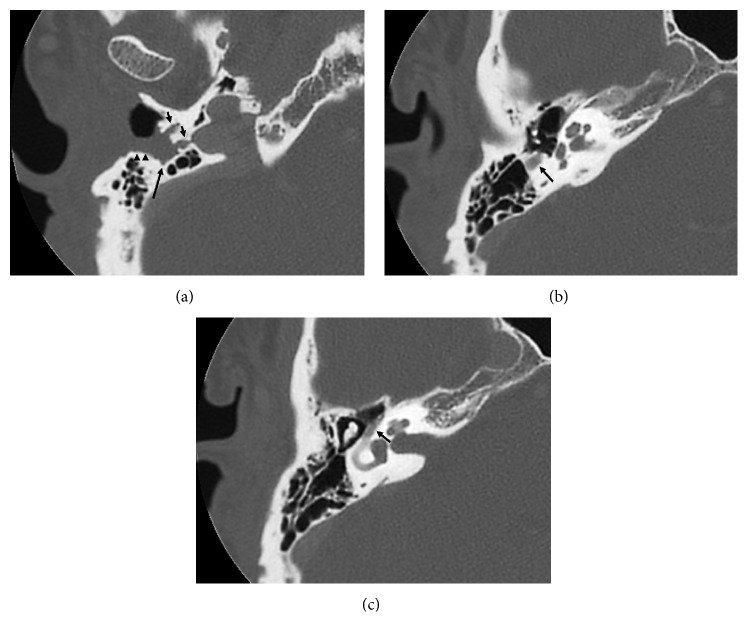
Axial CT images of the right temporal bone. (a) A mass in the stylomastoid foramen (large arrow) is destroying the tympanic bone and mainly spreading outside the bone (arrow heads). Note that, additionally, there is bony destruction on the inward side that might have spread along small branches of the facial nerve (small arrows). (b) The bony canal of the mastoid segment of the facial nerve is enlarged (arrow). (c) The bony canal of tympanic segment of the facial nerve is enlarged (arrow) as compared with the contralateral normal side (not shown).
